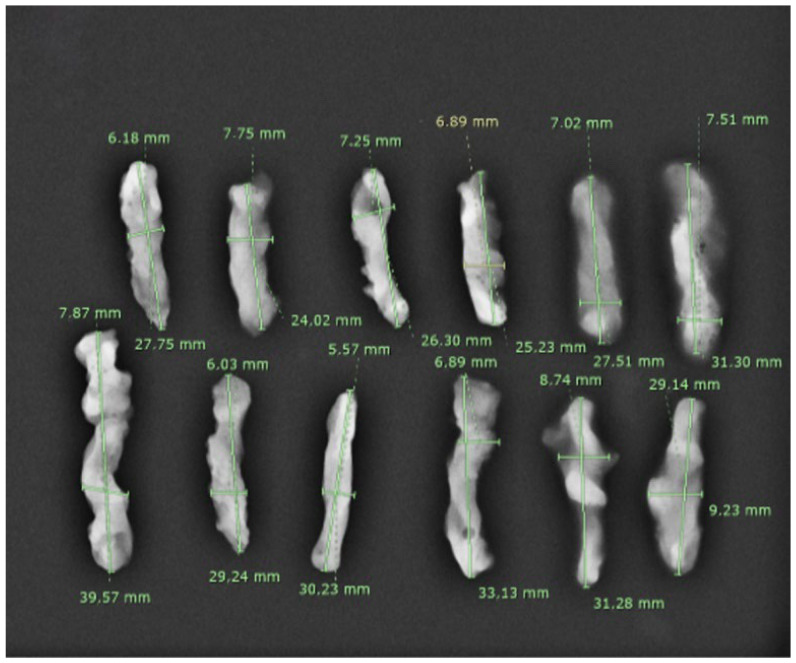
The X-ray scan of the fragments acquired using the Mammotome^®^ Revolve EX (aspiration level 5 and 25 mm window) with the measurements of each one.
